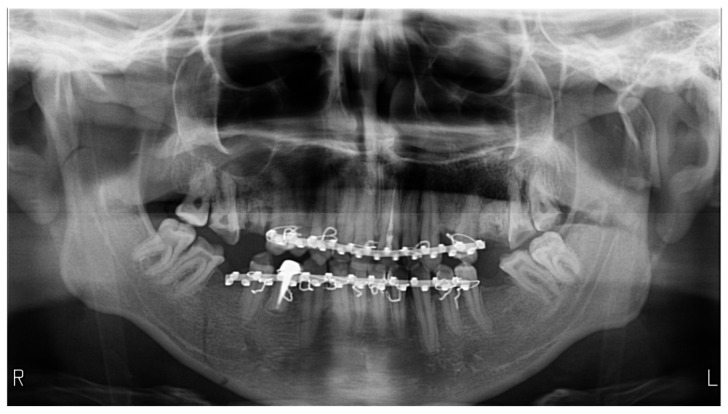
Panoramic radiography exemplifies the air present in the palatoglossal space as a radiolucent band. The patient presents a triple fracture of the mandible, bilateral low subcondylar and the body on the right side, with bimaxillary immobilization.
